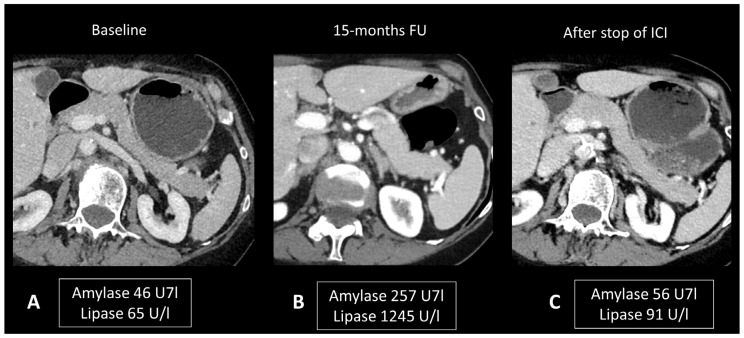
Immune-related pancreatitis in a patient with high-grade serous ovarian carcinoma treated by ipilimumab + nivolumab as the second line of treatment. Transverse CT images performed 15 months after initiation of treatment (B), due to elevated lipase and amylase levels, show a subtle diffuse enlargement of the pancreatic gland compared to the baseline CT scan (A). Note the regression of this enlargement after cessation of treatment and normalization of pancreatic enzymes (C).
